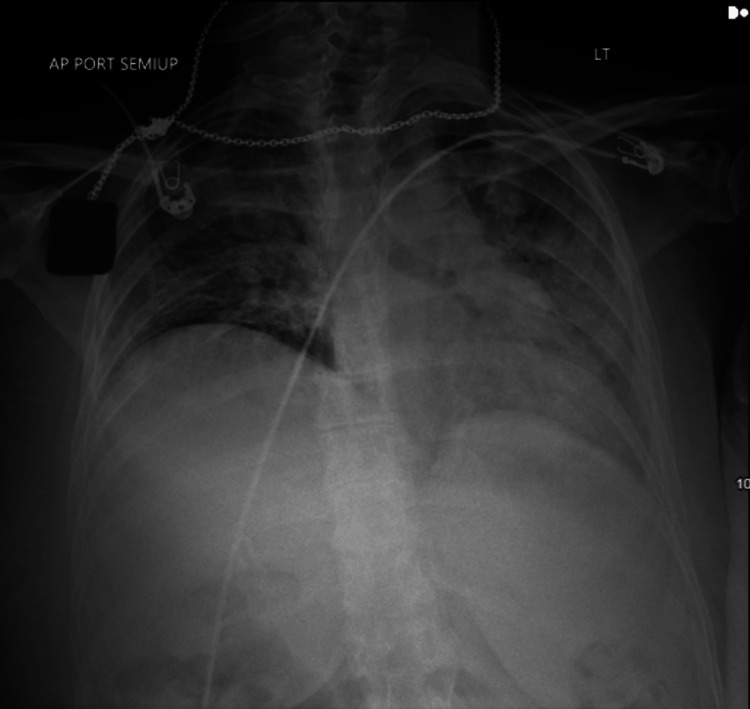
Anterior-Posterior Semi-Upright Chest X-ray Obtained Upon Admission
